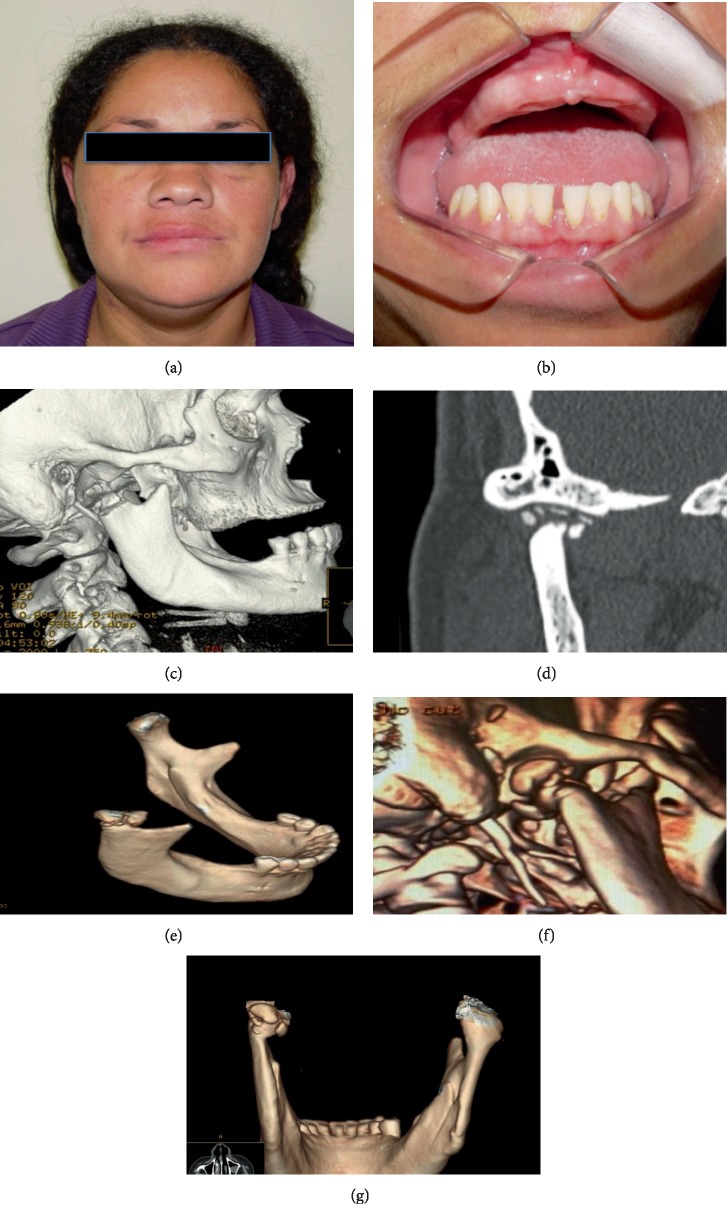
(a) Clinical aspect after surgery, with a slight improvement in facial asymmetry. (b) Mandible occlusal plane correction. (c) CT post resection image, without immediate reconstruction. (d) Tomography revealing the “islands of ossification” (arrows) 24 months of post surgery. (e, f) The confluence of ossification islands, with a “new condyle” formation after 3 years (arrows). (g) Image of a 5-year follow-up. The “new condyle” with a shape closer to a normal condyle (arrow).
